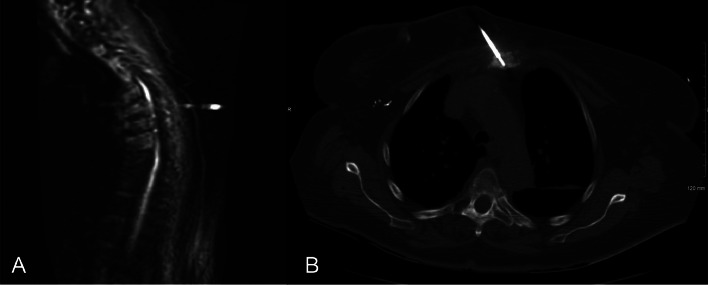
Biopsy images. (A) MRI bone biopsy showing location of sample retrieval at the T8 vertebral body. (B) Inferior manubrium CT bone biopsy of sclerosis.
